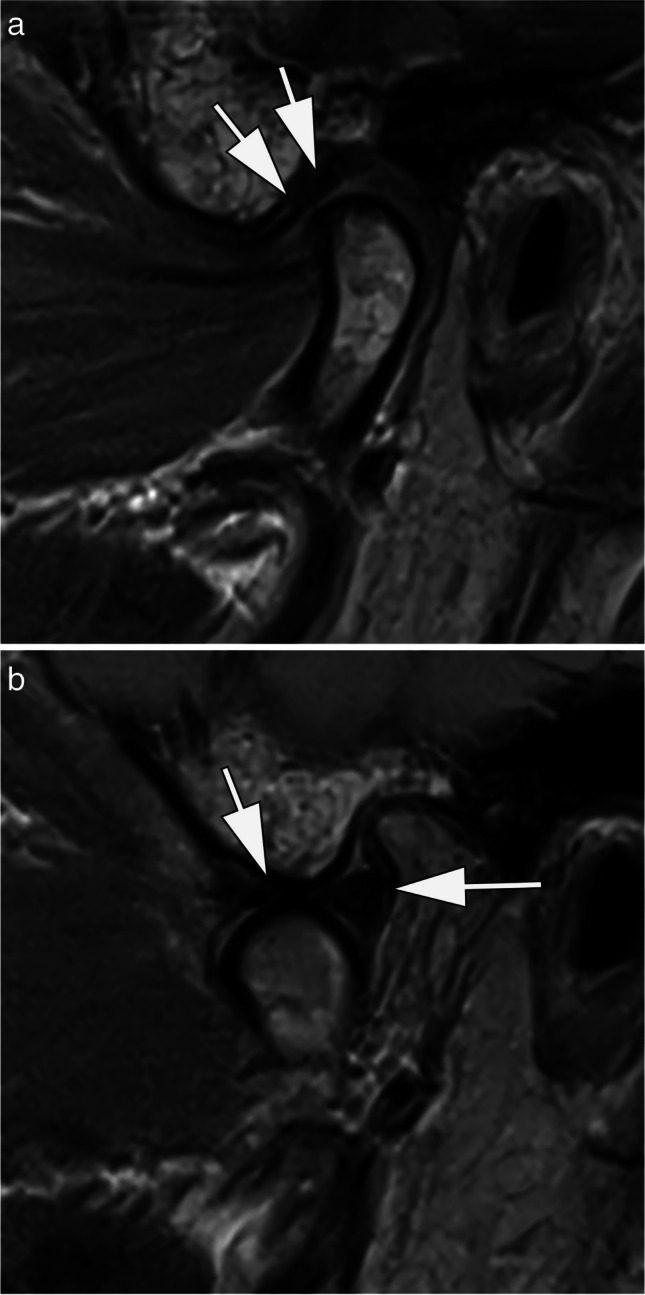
0.55-T MRI of the temporomandibular joint of a 55-year-old patient with chronic pain. A Sagittal proton density-weighted image with closed mouth shows a normal disc morphology and position without degenerative changes of the temporomandibular joint (arrows). B The open mouth position also shows a normal morphology and position of the disc (arrows). TA: 02:48 min (A), 02:48 min (B)
